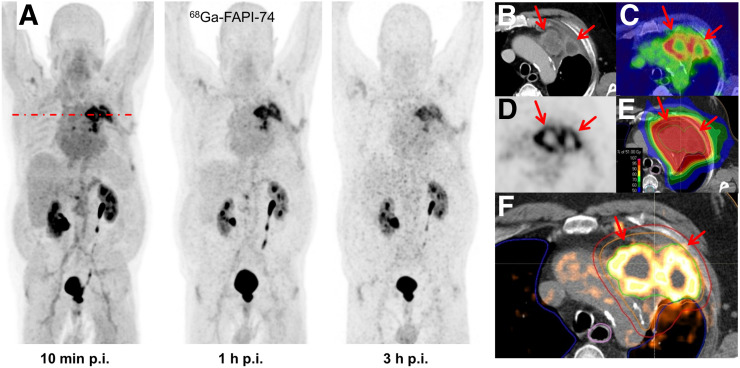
(A) Maximum-intensity projections of ^68^Ga-FAPI-74 PET at 10 min, 1 h, and 3 h after injection. (B–E) Direct comparison of contrast-enhanced CT (B), fusion imaging (C), and FAPI PET (D). (E and F) Superior tumor delineation consecutively improved dose application to tumor volume using volumetrically modulated arc therapy. Positive FAP uptake is marked by arrows (B–F). Green outline = GTV; orange outline = clinical target volume; red outline = planning target volume. p.i. = after injection.
